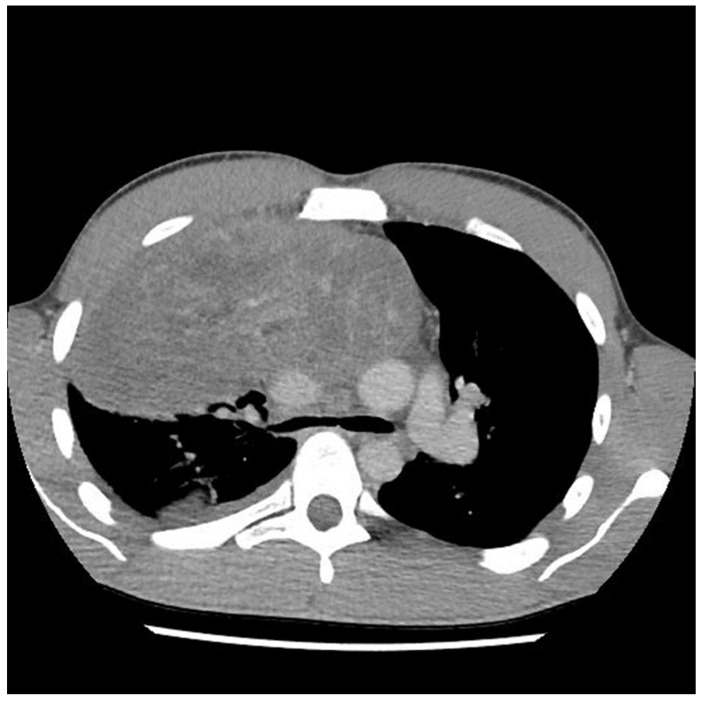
Computed tomography (CT) scan image of mediastinal mass at the time of initial presentation.
